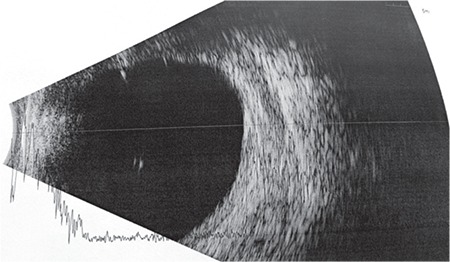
B-mode ultrasonography before surgical intervention
